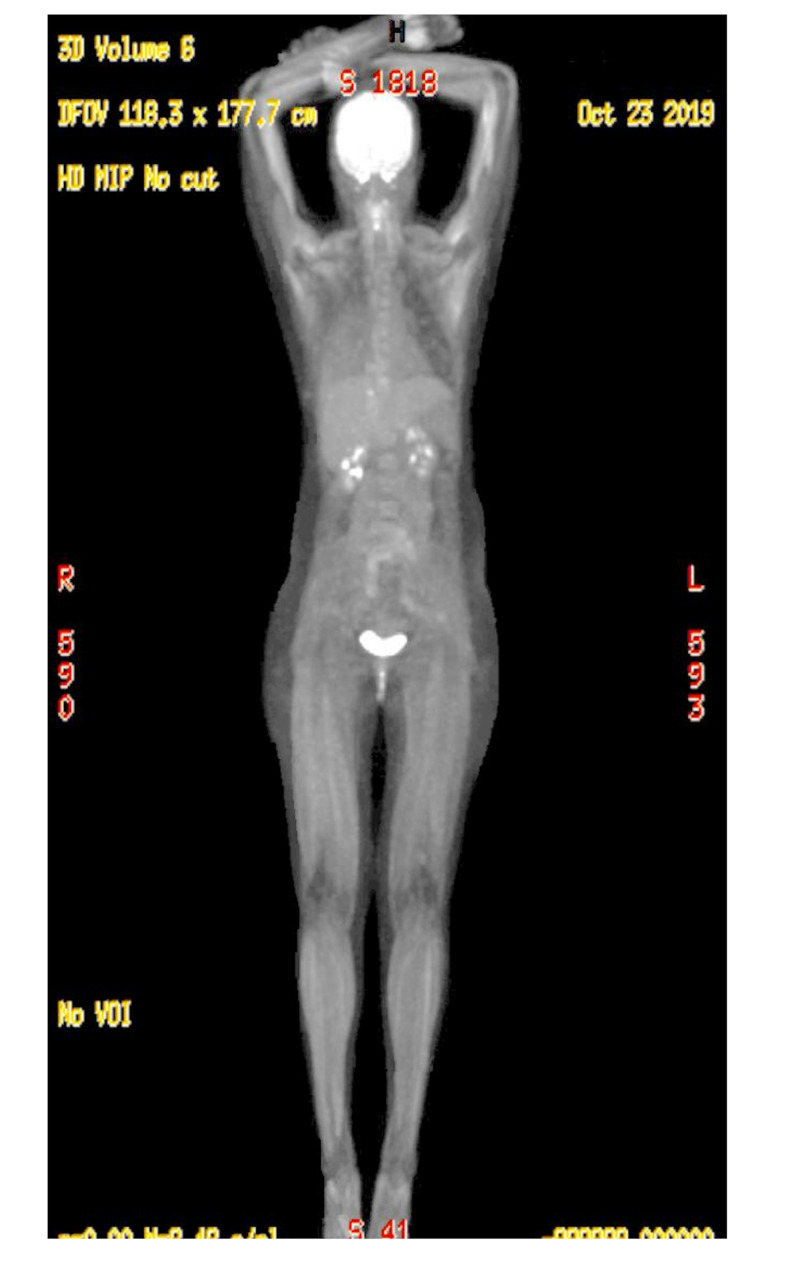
PET/CT after erdafitinib PET/CT, positron emission tomography/computed tomography
